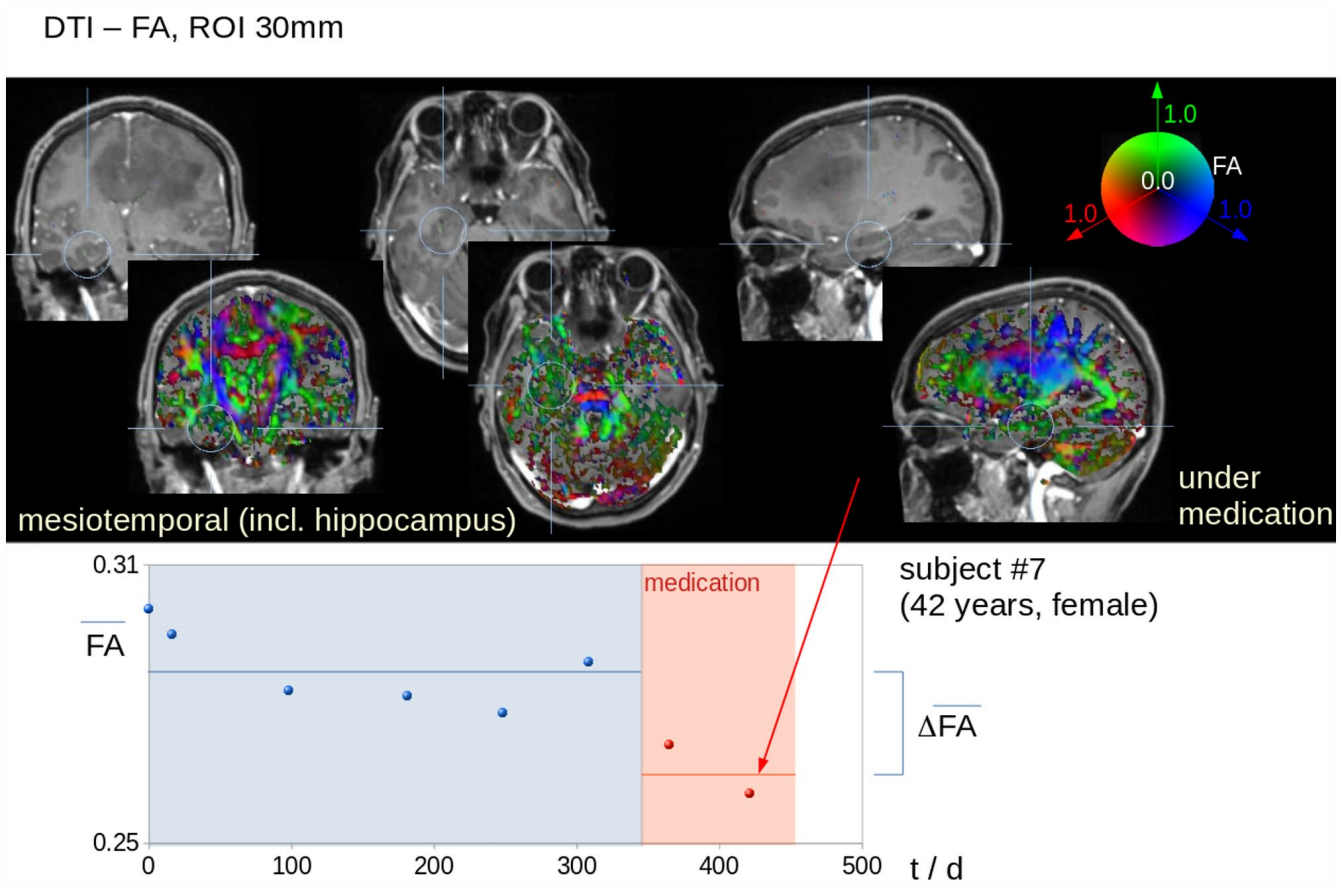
Individual example (patient #7, 42  years, female) for mesiotemporal (hippocampal) FA ROI analysis. Upper panel: ROI localization in the FA maps. The position of the mesiotemporal ROIs include the hippocampus as visualized in the T1-weighted images (contrast agent) and in the color-coded FA maps, that way focussing on brain areas in which a change reflecting a cognitive decline is hypothesized. The threshold of 0.2 (as applied in the color-coded FA maps) shows that gray matter as well as CSF was excluded from the analyses, that way focussing only on white matter alterations. Lower panel: Average FA values in the left mesiotemporal (hippocampal) ROI show a decrease under bevacizumab therapy.
